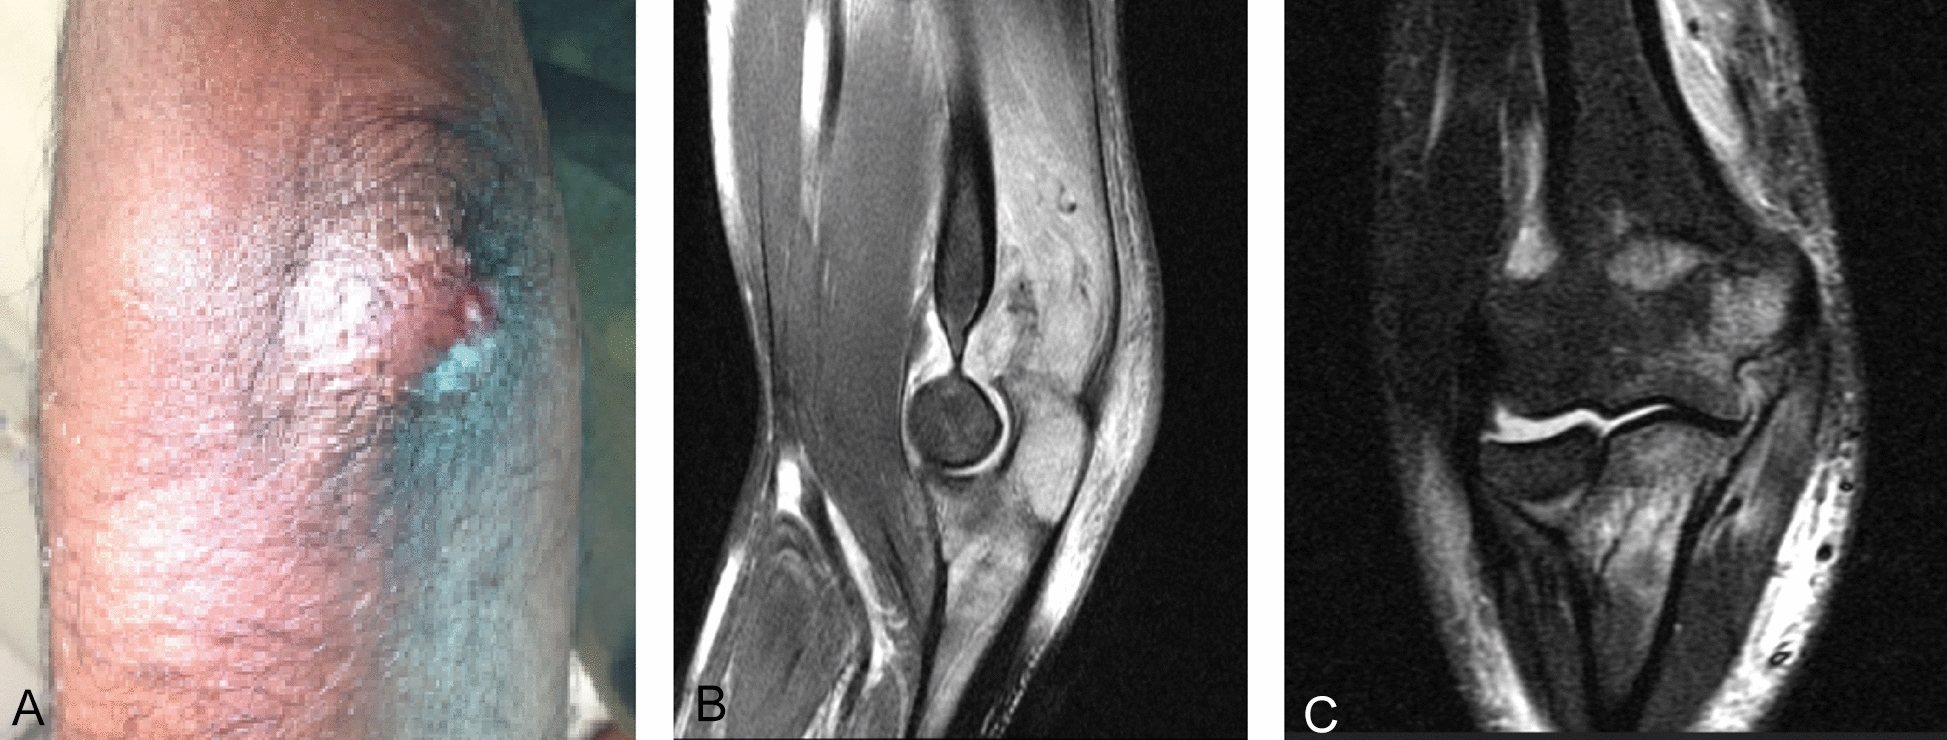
A Clinical impression of the olecranon (dorsal, left) on 25 June 2020; B and C magnetic resonance imaging scan (B, sagittal; C, coronal) with peripheral edema of the proximal olecranon as a sign of a beginning osseous infection
